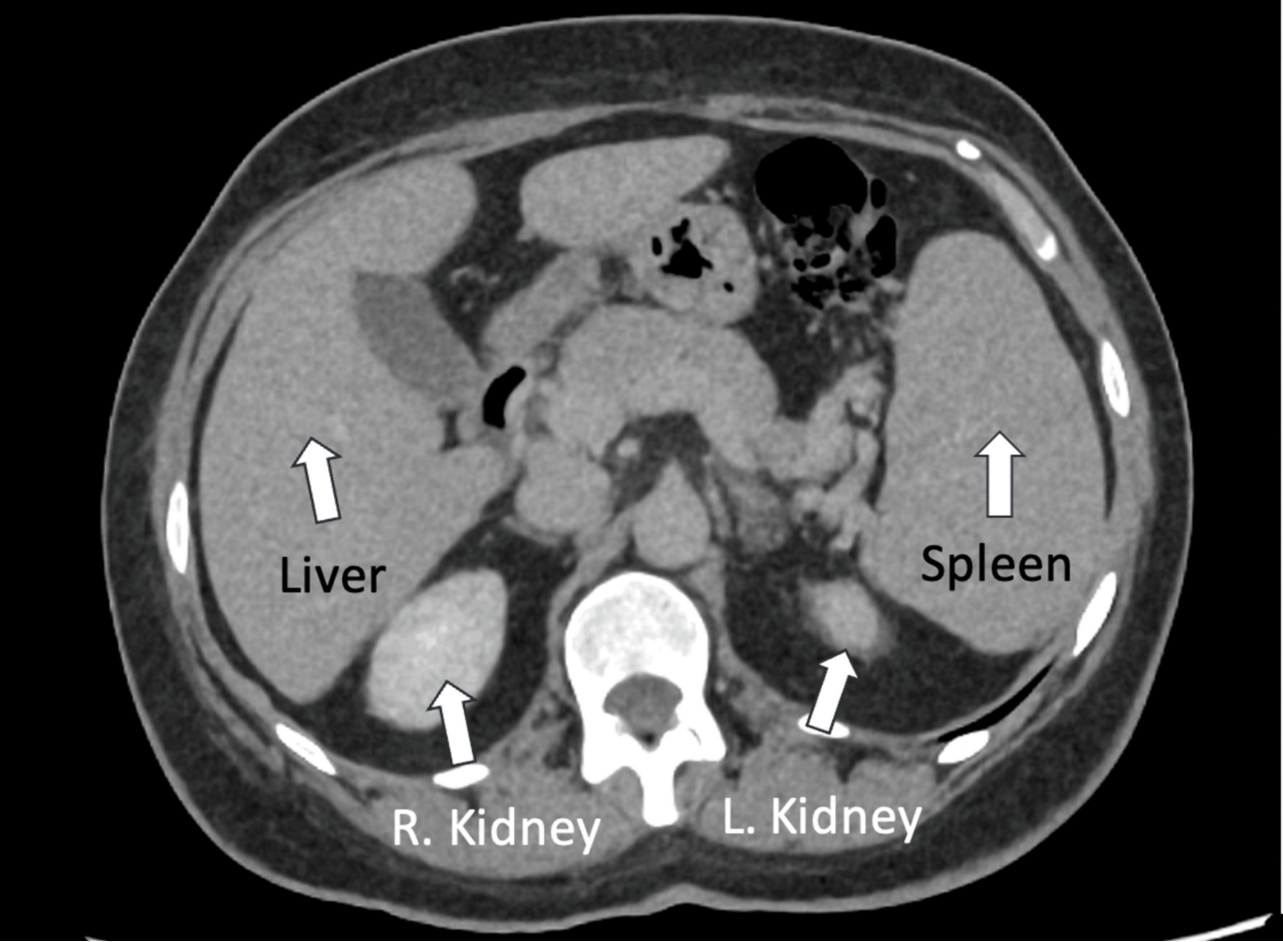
Axial CT abdomen demonstrating spleen mildly enlarged in size (14.7 cm span)
